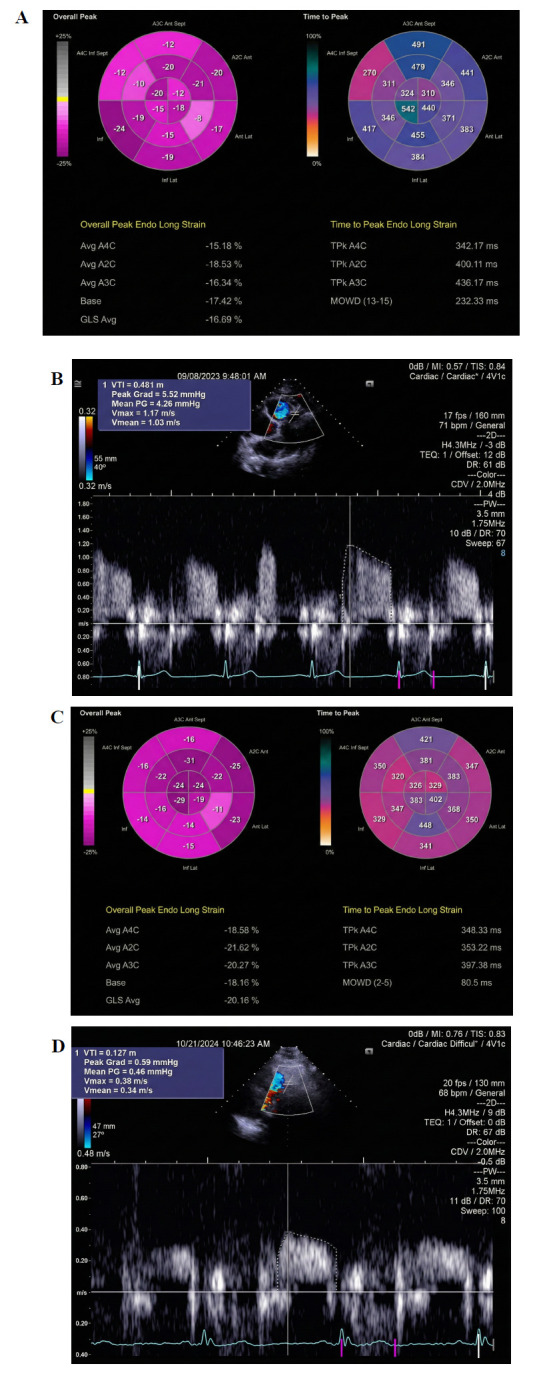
The presentation of 2D-STE and CA-USI plots for both patient groups. (A), 2D-STE bull's-eye plot of the observation group. (B), CA-USI plot of the same patient in the observation group. (C), 2D-STE bull's-eye plot of the control group. (D), CA-USI plot of the same patient in the control group. 2D-STE, two-dimensional speckle tracking echocardiography. CA-USI, coronary artery ultrasound imaging.
